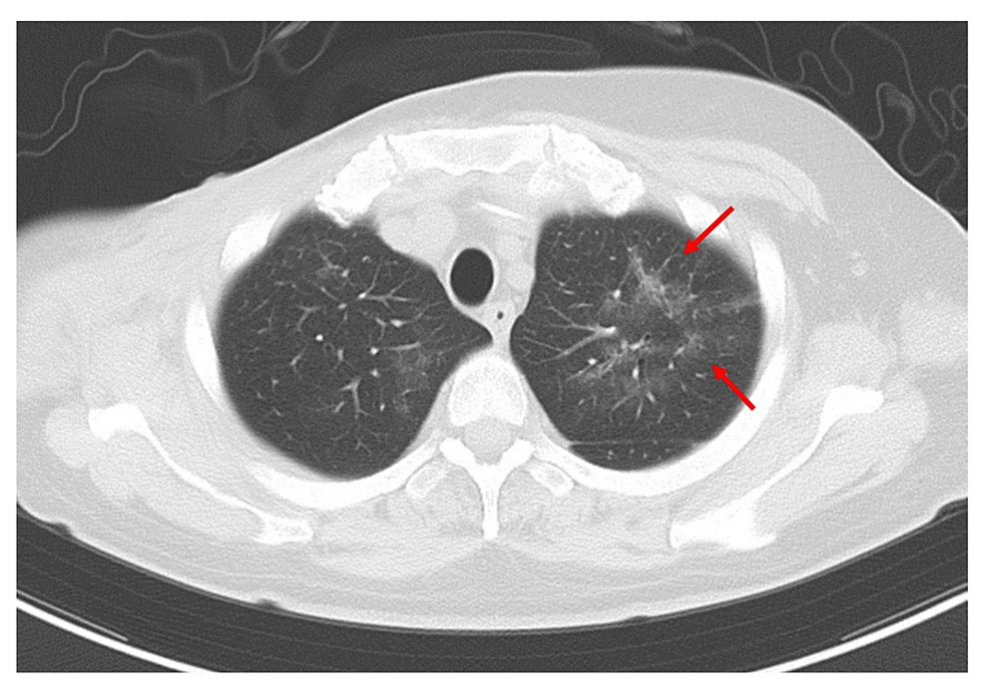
CT image of the patient on day 309. Compared with the imaging findings on day 289, the ground-glass opacities within the S1-2 segments of the left upper lobe were less conspicuous (arrows).
